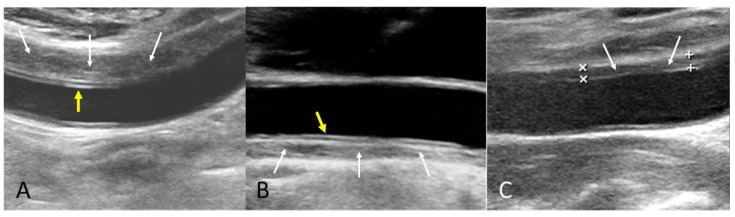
Intramural thrombus with train-line pattern (A,B) and without train-line pattern (C). The ultrasound image was obtained using a 4× magnification setting. Yellow arrows indicate the train line. White arrows indicate intramural thrombus. Symbols such as “×” and “+” were generated by the ultrasound machine and used by sonographers to mark ROIs.
